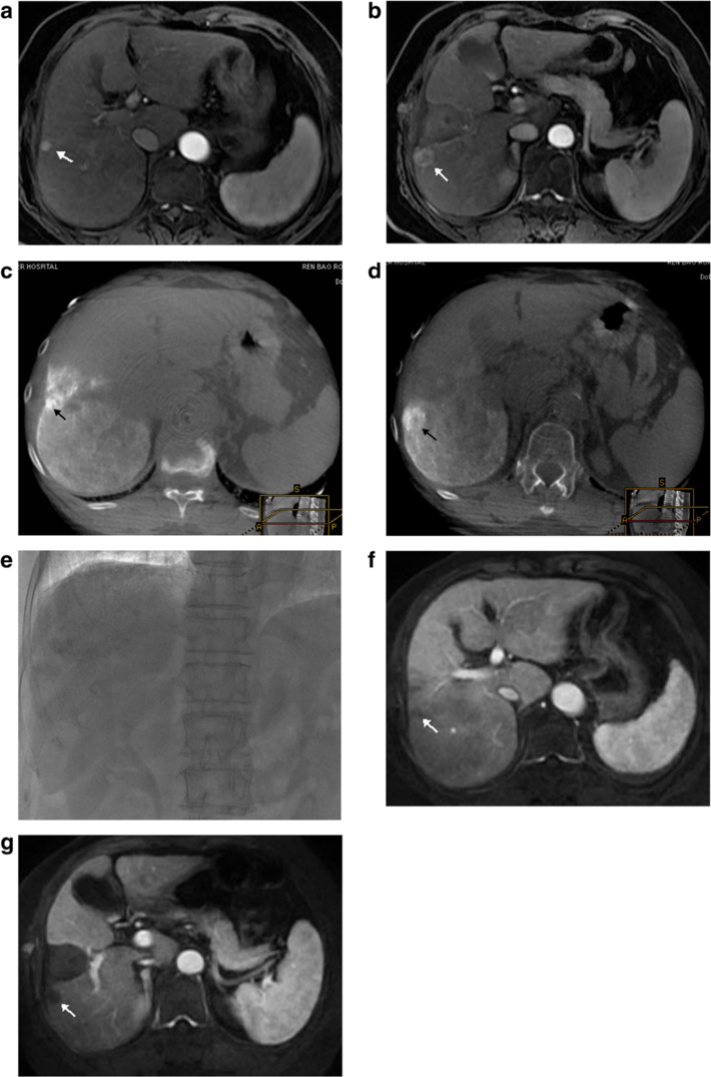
A 56-year-old female with multiple HCCs and prior history of radiofrequency ablation. a, b Preprocedure arterial phase contrast-enhanced MRI shows two tumors adjacent to the ablation margin in the right lobe (*arrows*); c, d Cone beam CT immediately after completion of TACE showed these two tumors with complete lipiodol retention pattern without defect concordant with MRI; e On the fluoroscopy image, tumor lipiodol retention was not clearly seen. f, g Postprocedure 1 month follow-up contrast-enhancement MRI showed necrosis without contrast enhancement in both tumors (arrows) representing complete response
